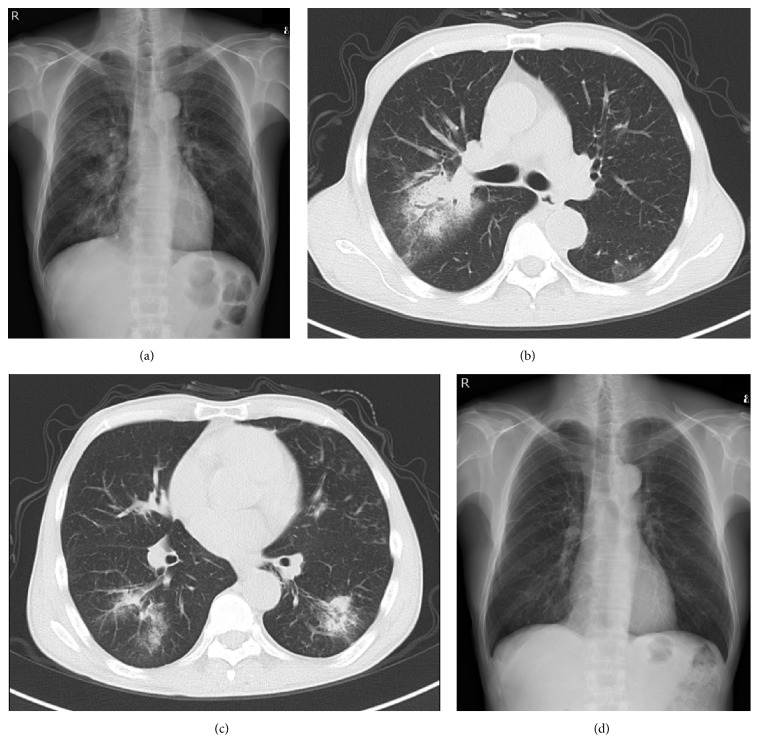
(a) Chest radiograph shows patchy bilateral consolidation in the middle lobe and bilateral lower lobes. (b, c) Chest computed tomography reveals patchy consolidation, ground glass opacities, and small nodular opacities in the middle (b) and bilateral lower lobes (c). (d) Follow-up chest radiograph after 3 weeks shows resolution of abnormal findings.
